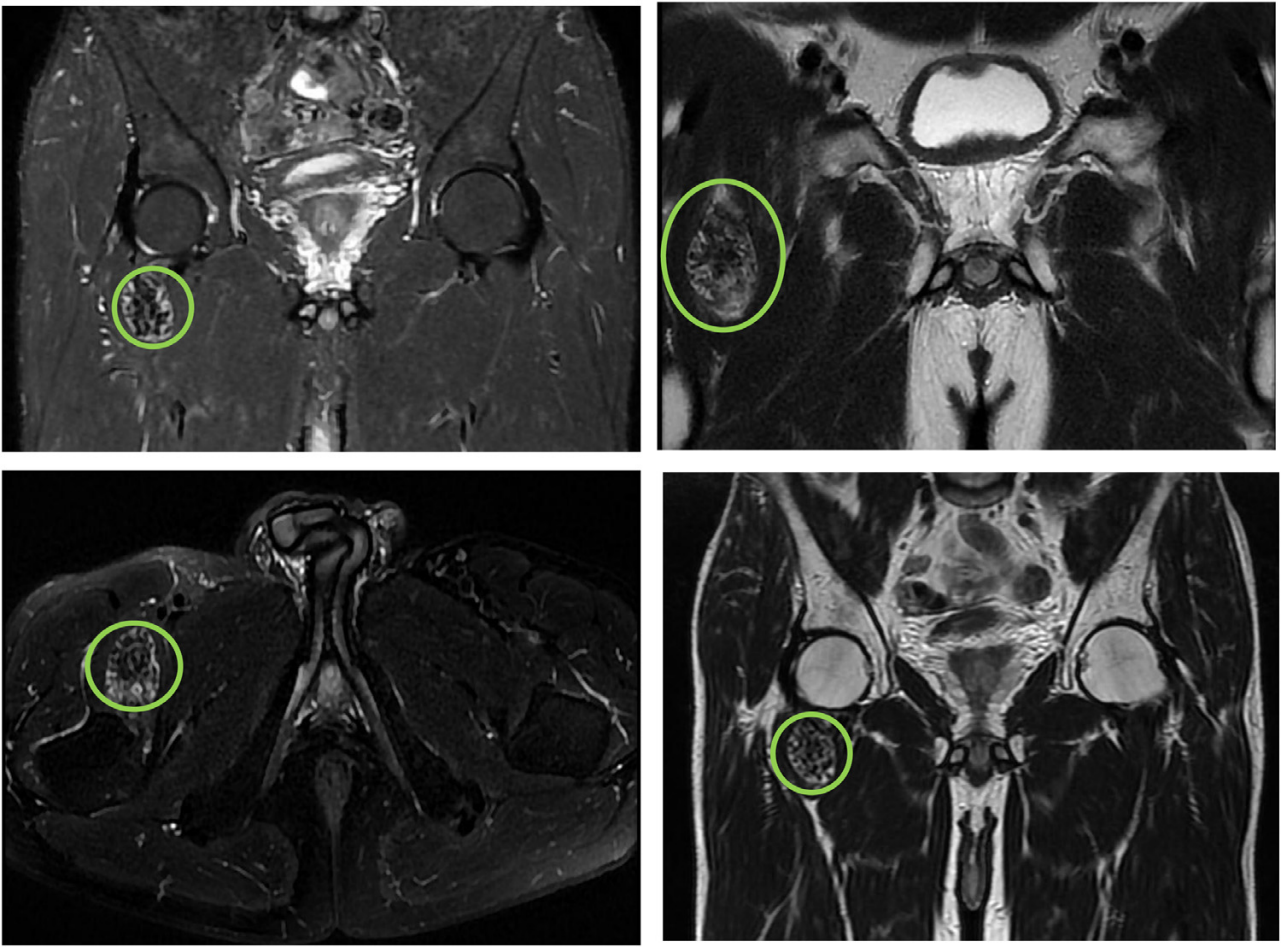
MRI of the pelvis on T2-weighted and fat sat axial and coronal image, the lesion shows a heterogeneous hypersignal with central areas of hyposignal (green circle).
